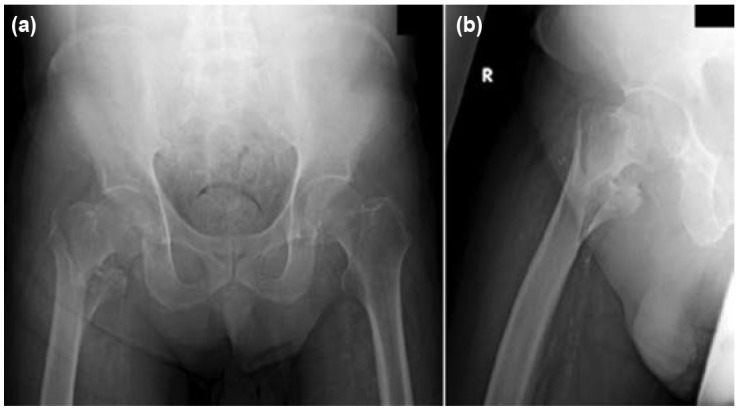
(a) Anterior-posterior view and (b) Lateral view of plain radiographs on admission showing a four-part displaced comminuted right intertrochanteric fracture.
